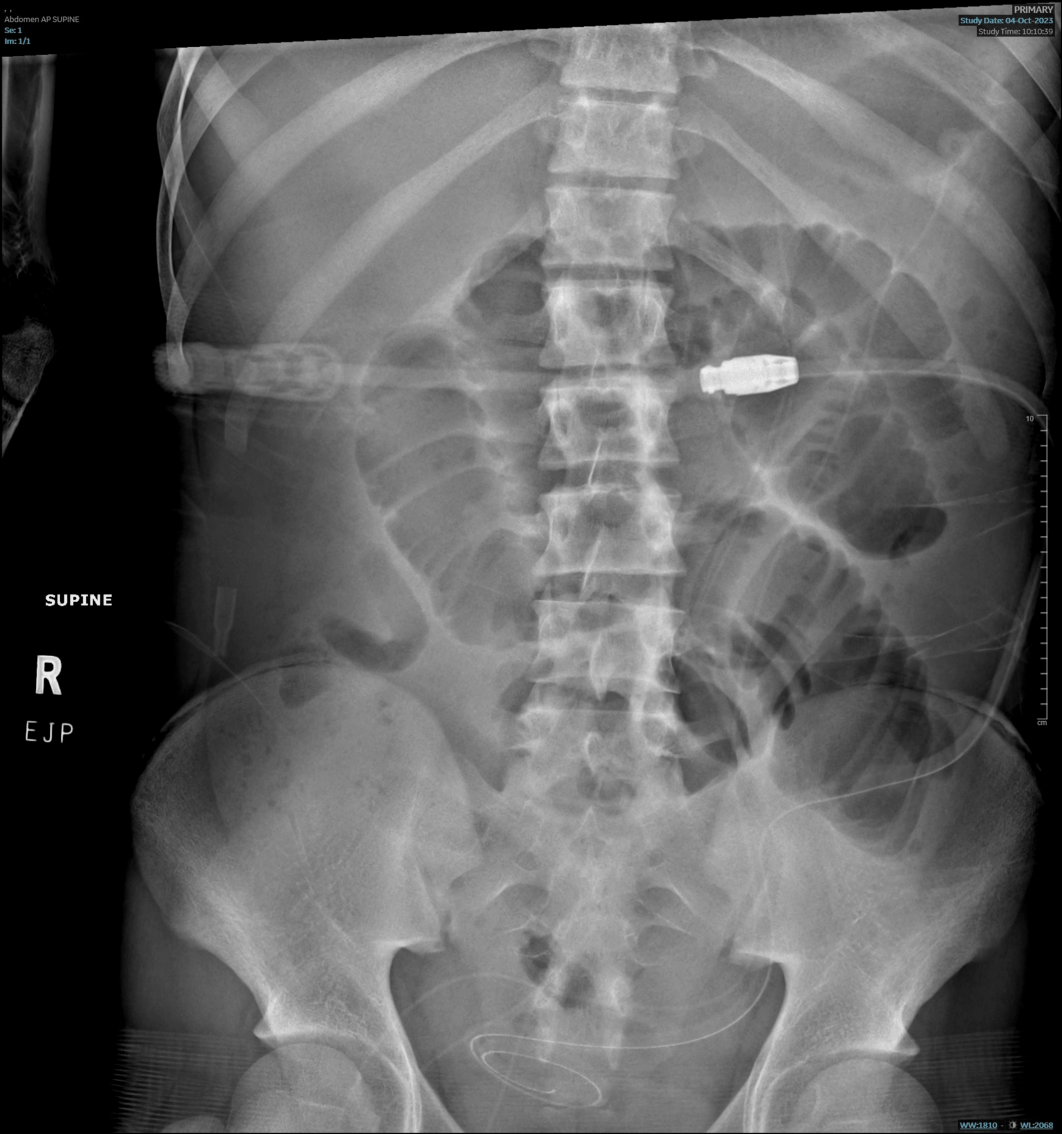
Abdominal x-ray showed distended centralized small bowel loops with air-fluid levels in a pattern typical for small bowel obstruction.
